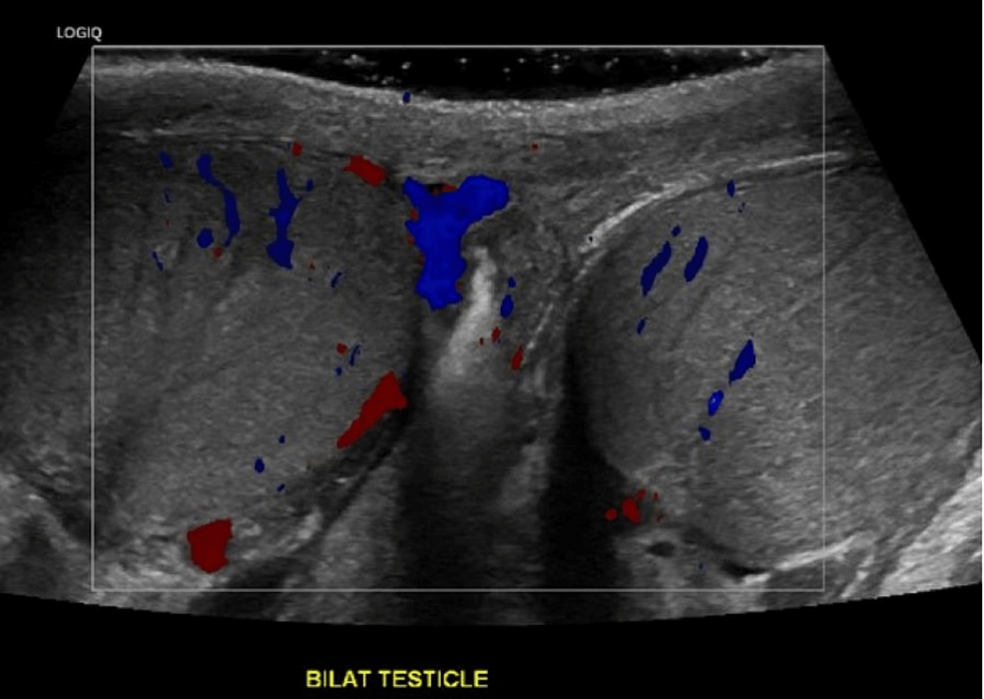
Testicular ultrasound showing homogeneous texture and normal Doppler flow
